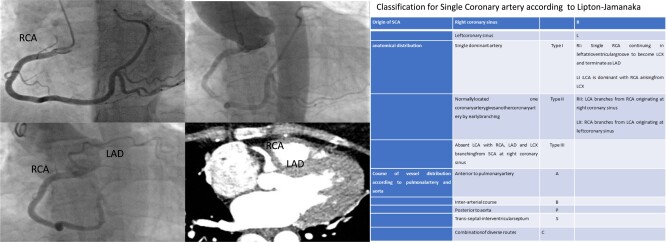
A 55-year-old female presented with dyspnoea and light chest pain during physical activity and palpitations. Coronary angiography and cardio-CT revealed a rare congenital anomaly: single dominant right coronary artery (RCA) with hypoplastic left coronary branch, which does not fit any of the types of the modified Lipton’s classification.
